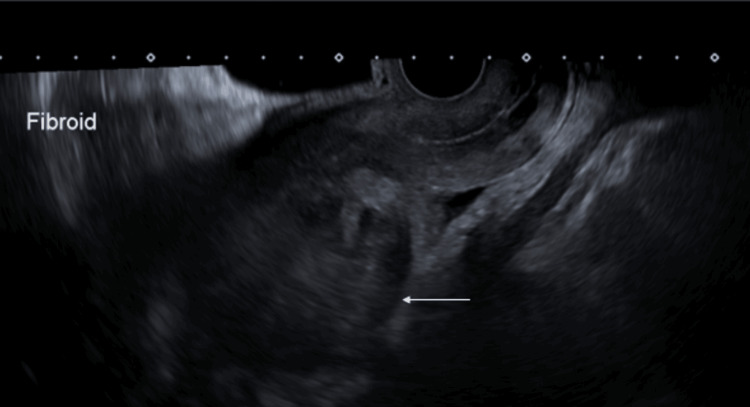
Pre-embolisation transvaginal ultrasound image in the sagittal plane depicting a fundal fibroid (arrow)
